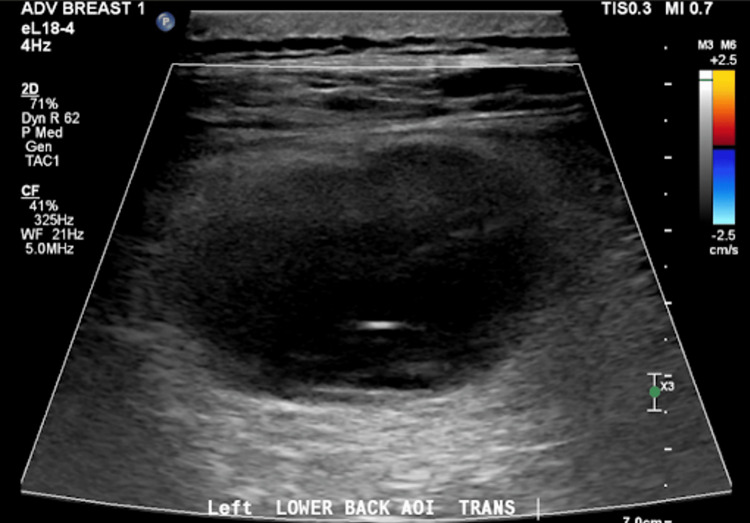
Ultrasound showing mass with complex cystic components
